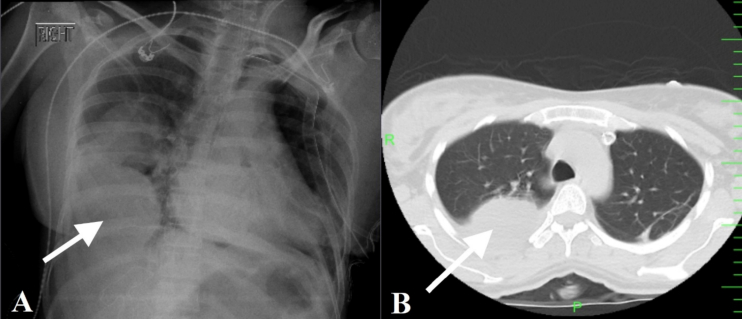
This figure shows a pulmonary mass in the lower middle segment of the right lung on chest radiograph (A) and CT scan (B).
